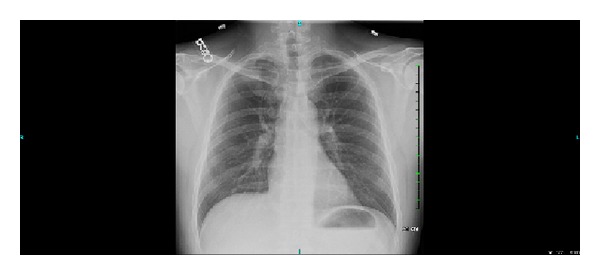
Chest X-ray of our patient before right first rib resection.
